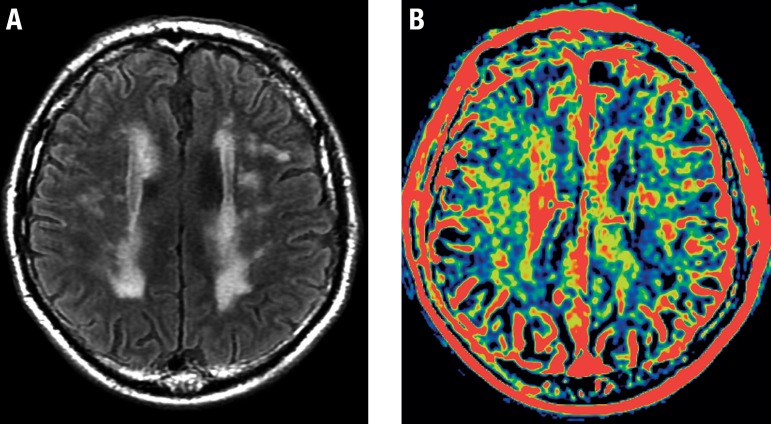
BBB permeability changes in SVD. A 76-year-old male with SVD. Axial FLAIR image (A) shows extensive and confluent white matter hyperintensities of presumed vascular origin. Color map proportional to wash-in rate (B), obtained from a dynamic contrast-enhanced T1 sequence, reveals presumed changes in BBB permeability (in yellow) that show some correspondence with FLAIR hyperintensities.
